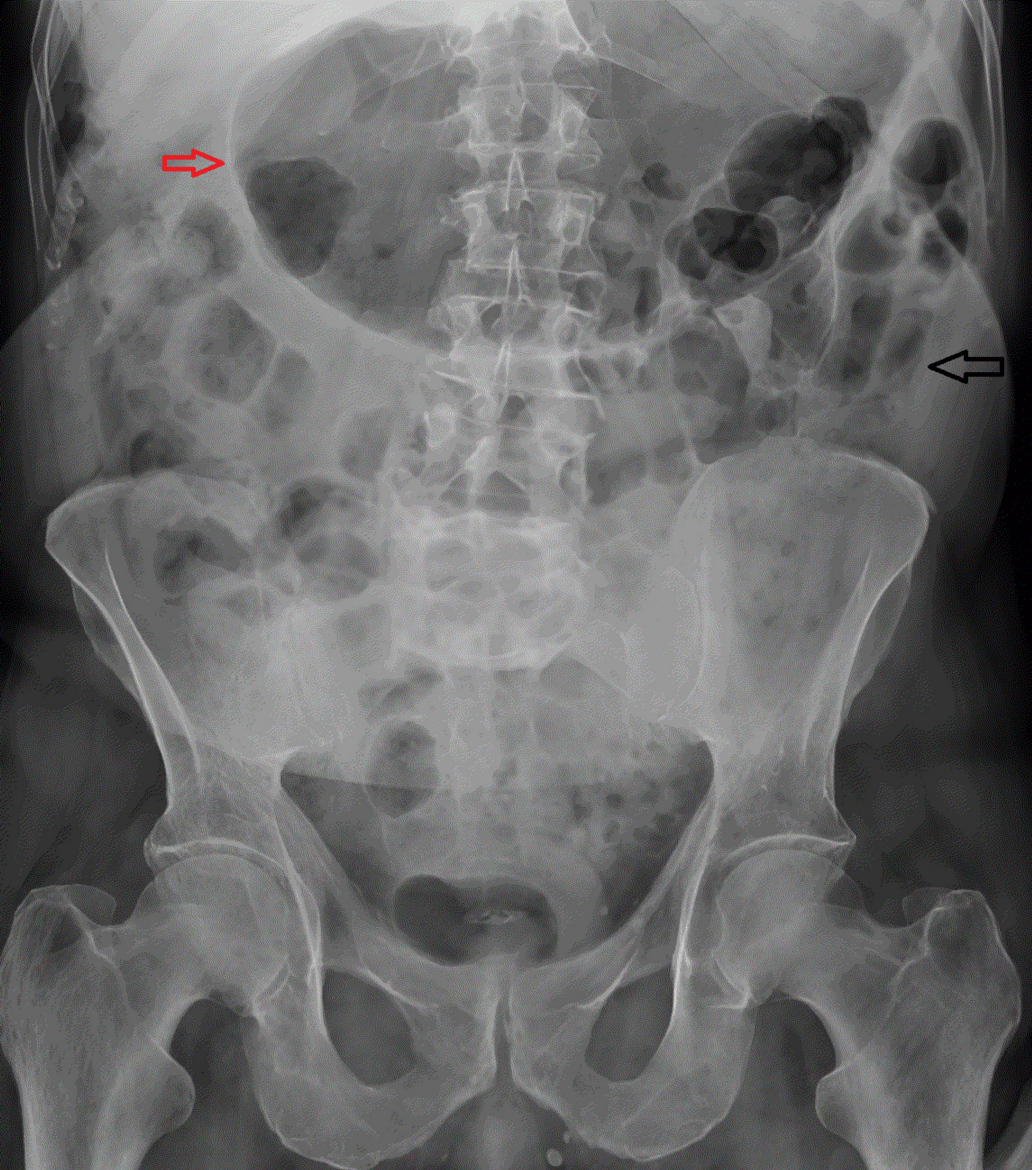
Follow-up abdominal X-ray. The black arrow shows interval improvement in the transverse colon and the red arrow shows the gassy stomach.
